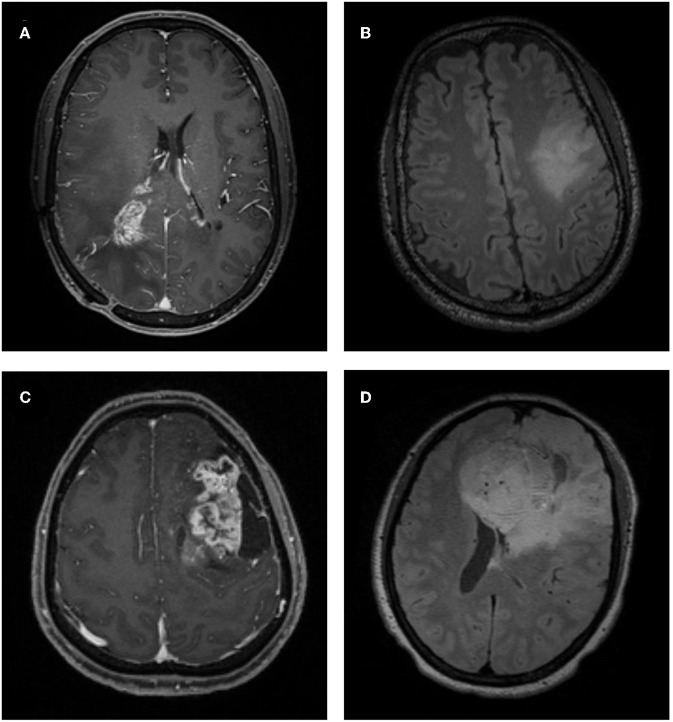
Examples of atypical low-grade oligodendroglioma and anaplastic oligodendroglioma on MRI. (A) A patient with atypical low-grade oligodendroglioma in contrast-enhanced T1-weighted (T1C) image. (B) A patient with atypical low-grade oligodendroglioma in fluid-attenuation inversion recovery (FLAIR) image. (C) A patient with anaplastic oligodendroglioma in T1C image. (D) A patient with anaplastic oligodendroglioma in FLAIR image.
